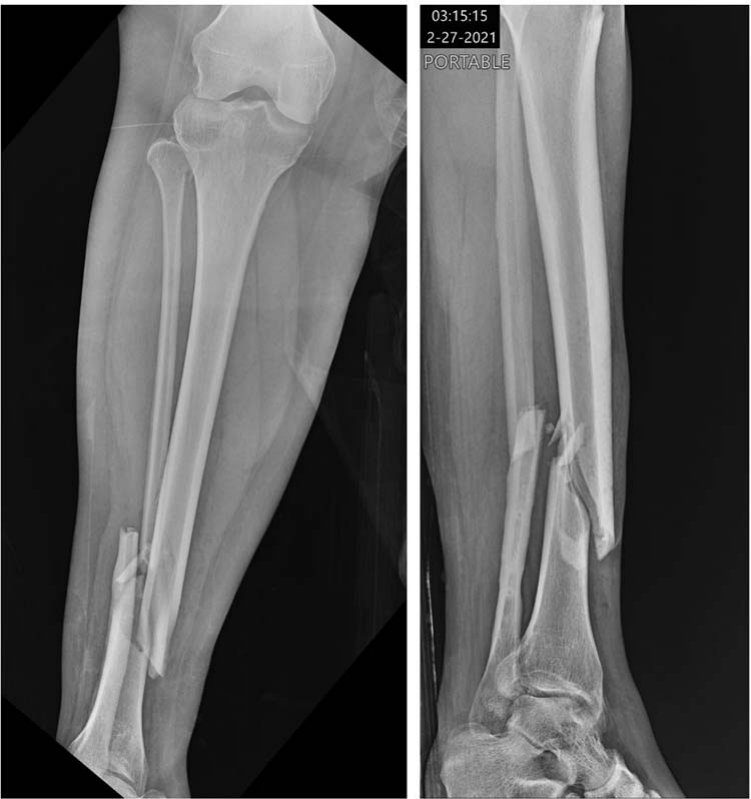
Initial radiographs demonstrating fractures of the proximal fibular neck and distal tibia and fibula.
